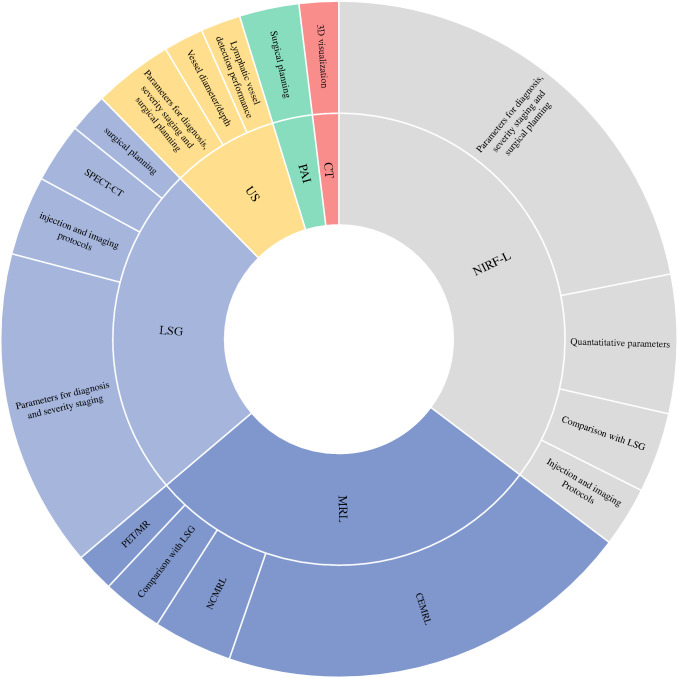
Overview of the included studies and their contribution to the lymphatic vessel imaging field. *LSG* lymphoscintigraphy, *US* ultrasound, *PAI* photoacoustic imaging, *CT* computed tomography, *NIRF-L* near-infrared fluorescence lymphography, *MR* magnetic resonance, *MRL* magnetic resonance lymphography, *CEMRL* contrast-enhanced MRL, *NCMRL* non-contrast MRL, *PET* positron emission tomography, *SPECT* single photon emission computed tomography
